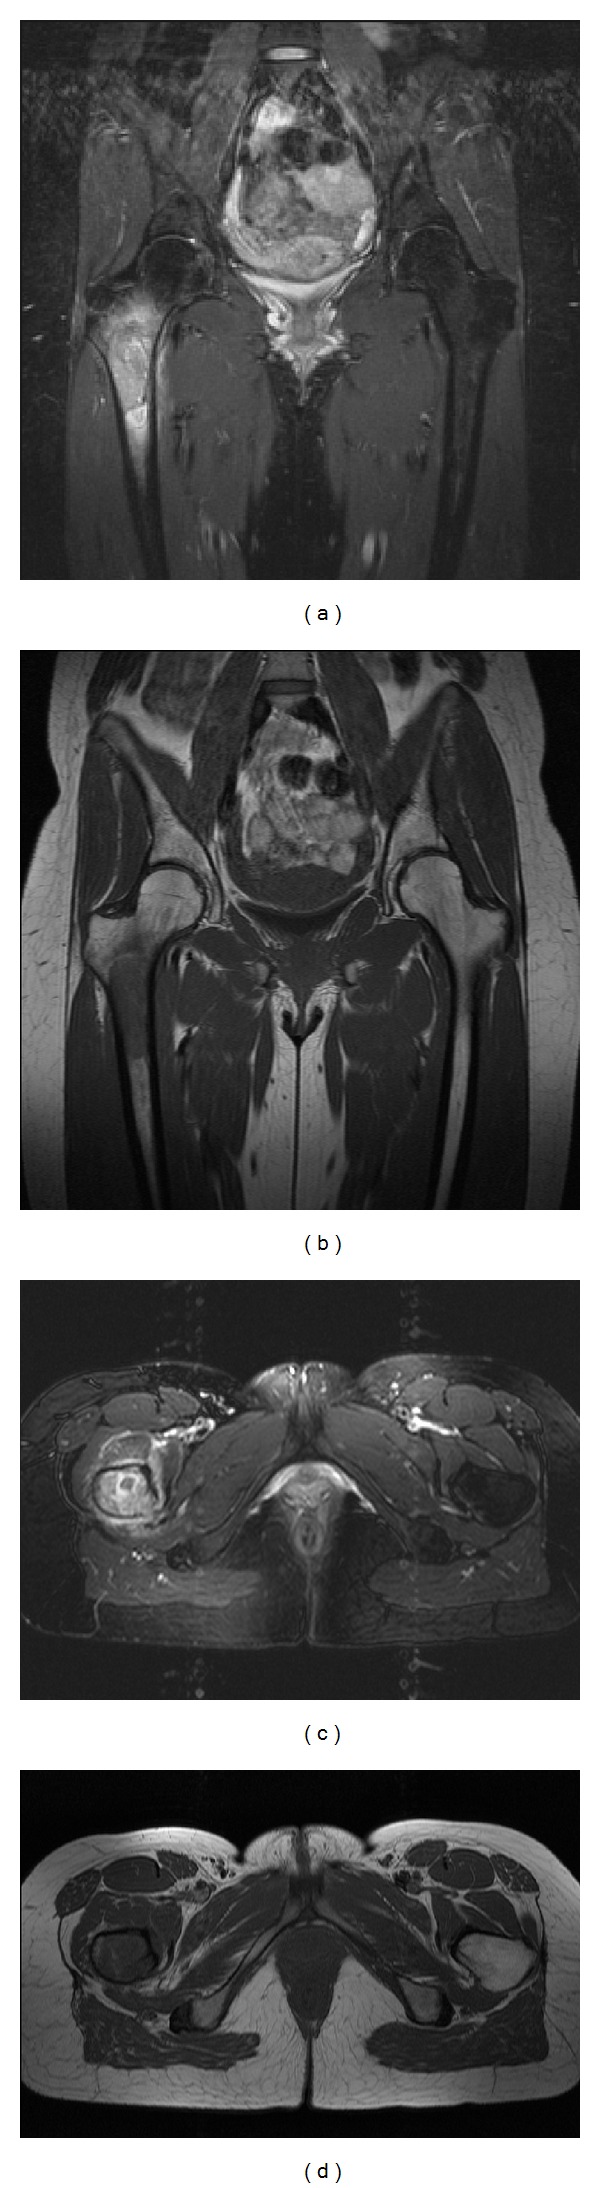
Unenhanced MRI of the right hip reveals a hyperintense lesion on STIR sequence ((a) coronal and (c) axial) and a correspondingly hypointense lesion on TI-weighted sequence ((b) coronal and (d) axial) with minimal cortical bone changes and no soft-tissue mass.
